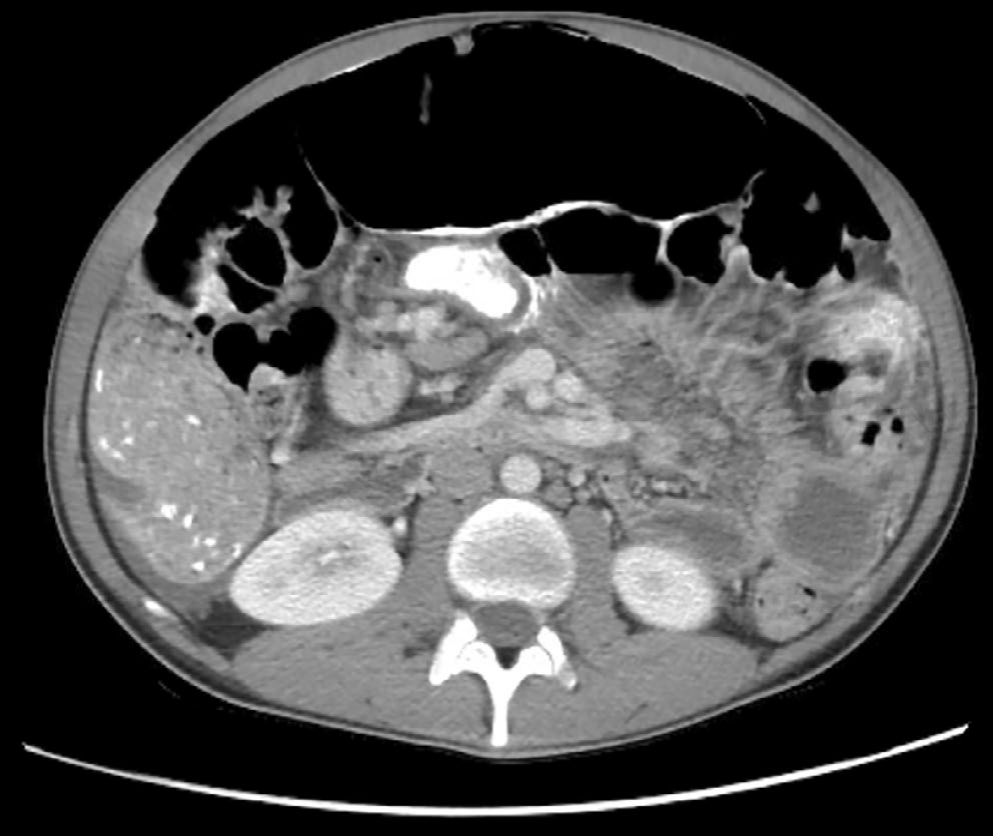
Transverse cross‐section of abdominal CT without contrast revealing distended loops of bowel.
